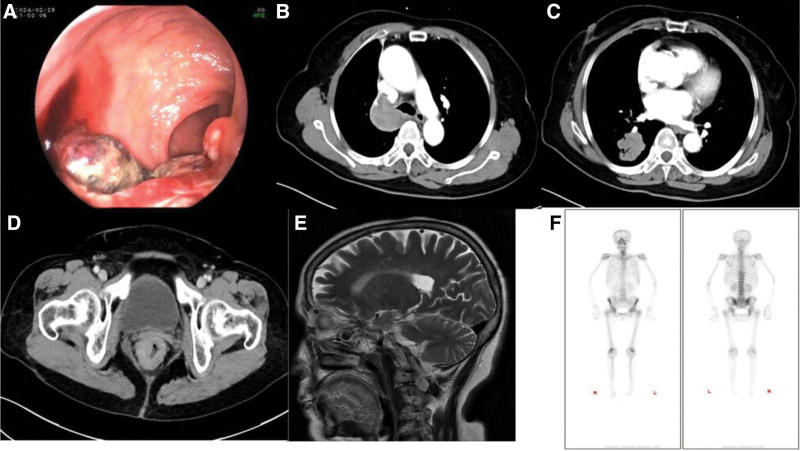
Electronic rectoscopy showed rectal tumor (A). Enhanced chest computed tomography (CT) scan revealed the neoplasms in the right upper lobe with a mediastinal lymph node metastasis (B, C). Enhanced abdominal CT showed rectal tumor (D). Cerebral magnetic resonance imaging (MRI) and bone emission CT (ECT) revealed no metastasis (E, F). CT = computed tomography, ECT = emission computed tomography, MRI = magnetic resonance imaging.
